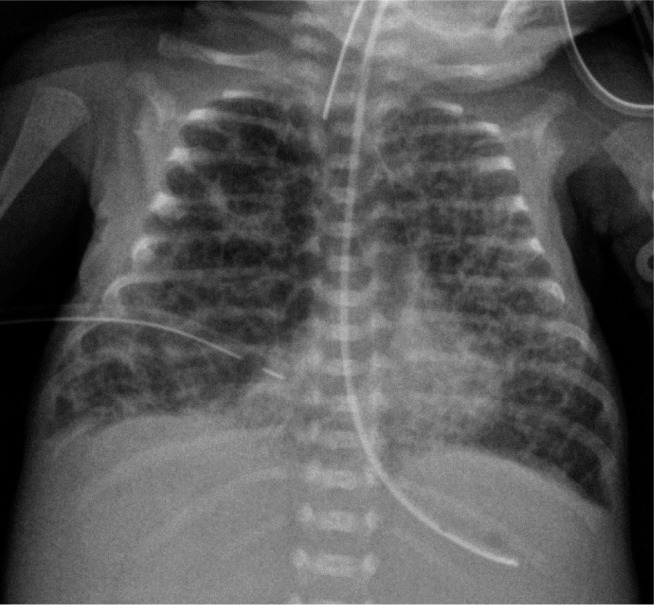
AP chest radiograph 1 hour after fibrin glue application via drain shows resolution of right pneumothorax. Persistent diffuse bilateral PIE. The left lung is better aerated than previously. A fibrin glue clot at the tip of the drain is observed.
